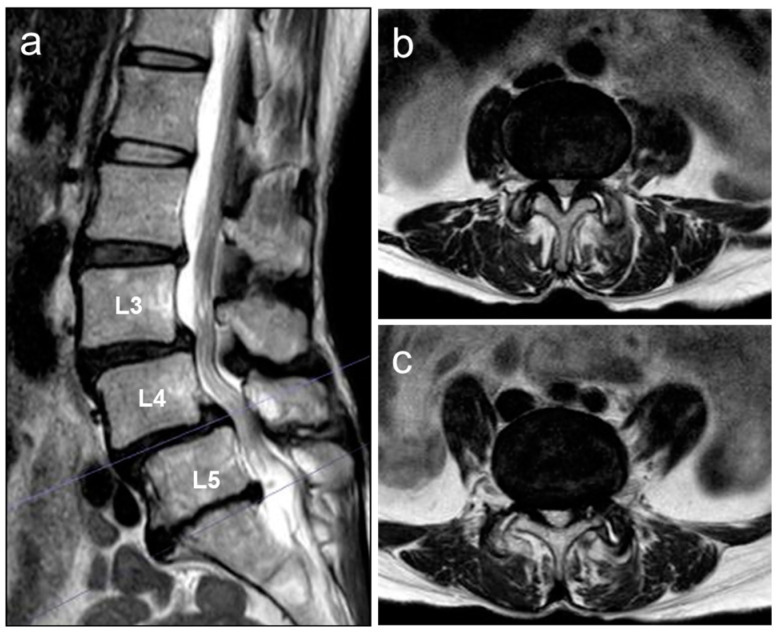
T2-weighted magnetic resonance imaging of the lumbar spine at baseline. (a) Midsagittal section, (b) axial section at L3/L4, and (c) axial section at L4/L5.
